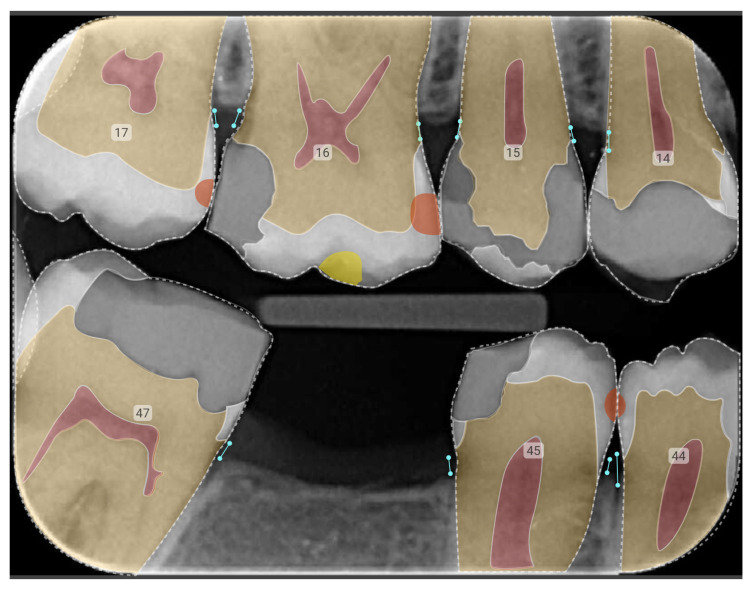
Example of annotated dental X-rays highlighting bone loss (turquoise lines) and radiolucency (orange semicircles). The numbers indicate the tooth numbers based on the FDI notation system.
